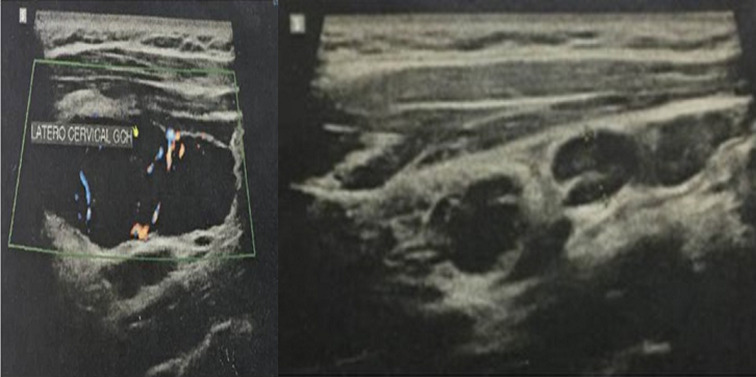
ultrasound images of cervical lymph nodes located in left IIB and V levels
